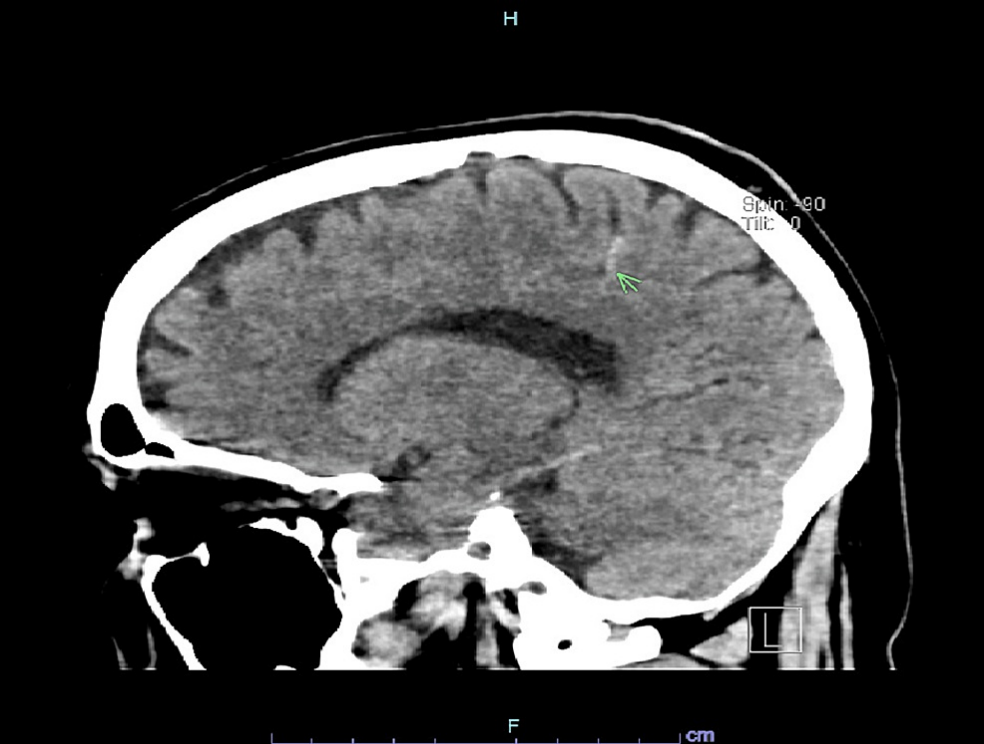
CT Head CT head showing evidence of subarachnoid hemorrhage (arrow)
